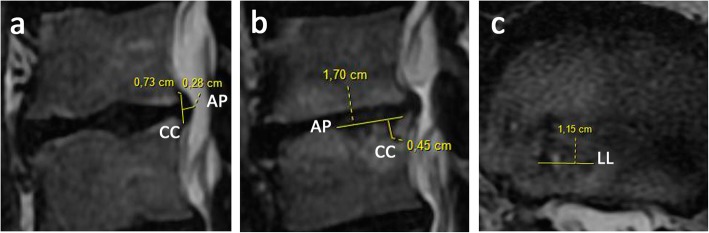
Axial and sagittal T2-weighted images (T2WI) 6 months post-treatment at the lumbar spinal level L3/L4 showing MRI measurements (in mm). a Sagittal measurements of lumbar disc protrusion diameter at cranio-caudal (CC) and antero-posterior (AP) sections; b sagittal dimension of SND at cranio-caudal (CC) and antero-posterior (AP) sections; and c axial dimension of SND at latero-lateral (LL) section
